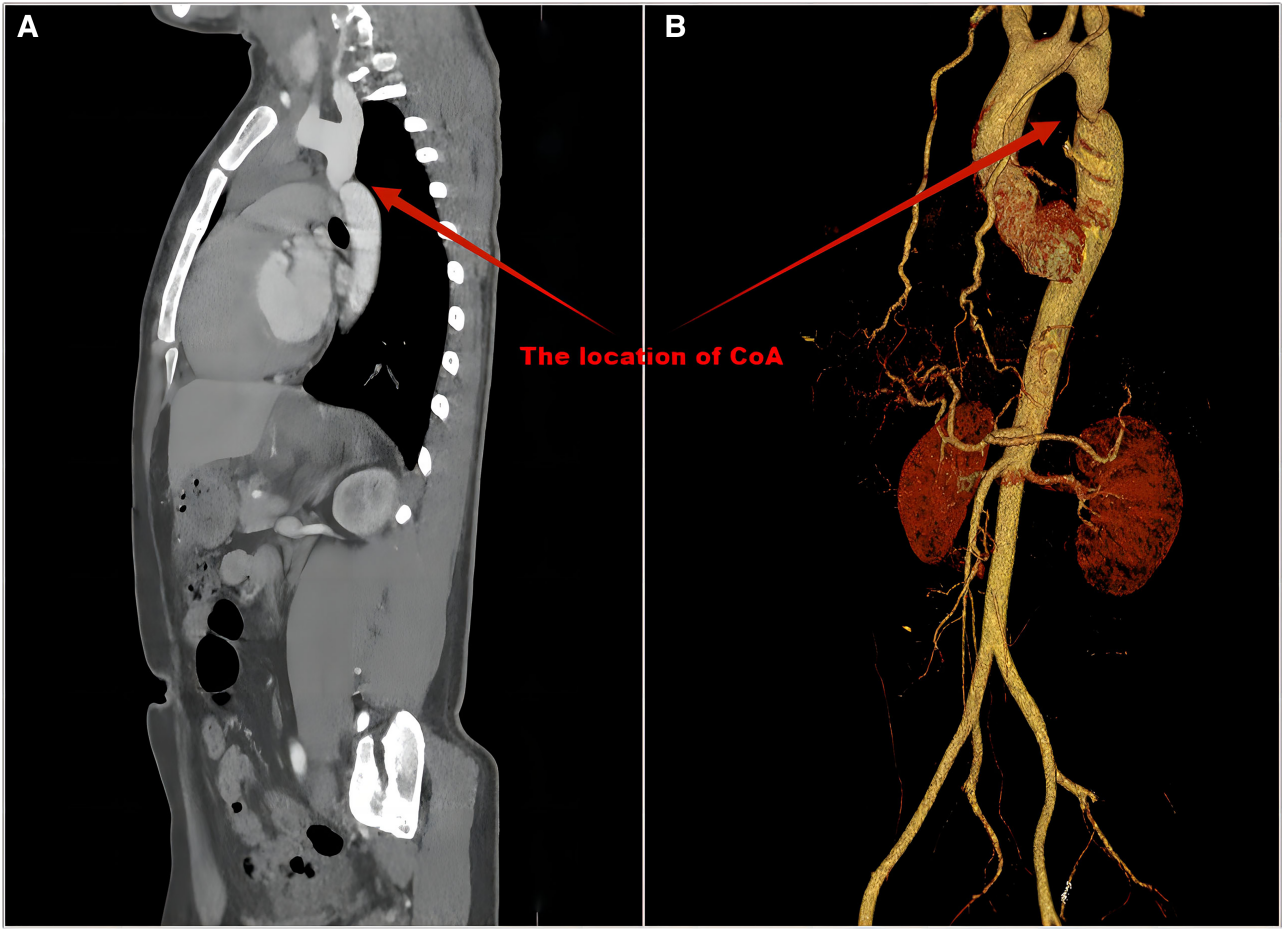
CT (A) and 3D reconstruction (B) of CoA. The precise location, diameter and length of the narrowing can be shown.
